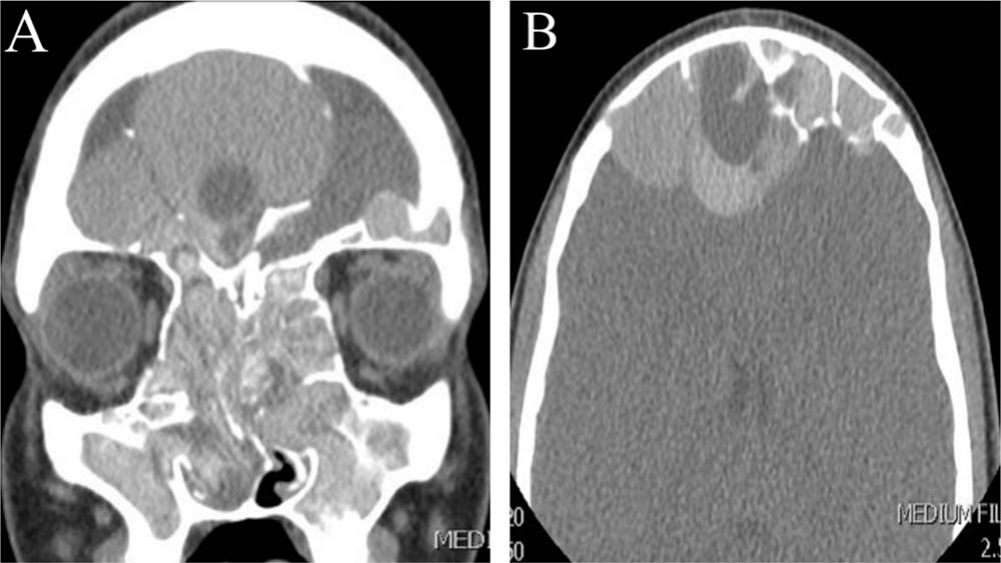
CT scan of paranasal sinuses in (A) coronal and (B) axial views, showing extensive disease with enlargement of sinuses, with more expansion of the right frontal sinus.
